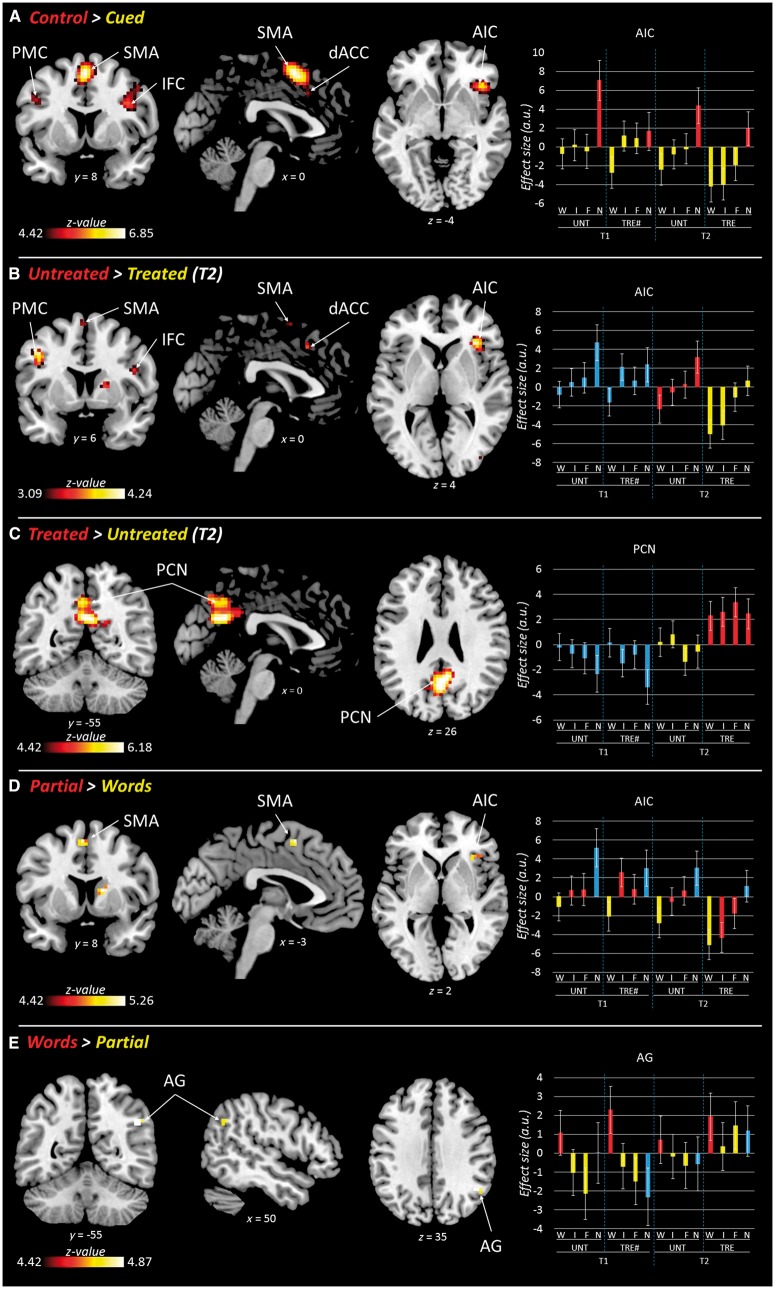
Functional MRI results. (A) Reductions in BOLD response related to immediate facilitation of naming. (B) Reductions in BOLD response related to long-term facilitation of naming. (C) Increased BOLD response for treated (as compared to untreated) items. (D) Activations related to partial (i.e. initial and final phonemes) cues processing. (E) Activations related to whole word cues processing. Results are displayed at *P* < 0.05 (FWE-corr.), except in (B), where they are displayed at *P* < 0.001 (unc.) for cluster extent, without correction at cluster-level (to allow for small-volume correction). Red and yellow bars refer to the conditions compared (red > yellow, as in the title of each contrast). Blue bars are conditions not included in the contrasts. a.u. = arbitrary unit; AG = angular gyrus; AIC = anterior insular cortex; Control = control trials (i.e. noise); Cued = cued trials (i.e. word, initial, and final); dACC = dorsal anterior cingulate cortex; F = final; I = initial; IFC = inferior frontal cortex; N = noise; Partial = partial cues (i.e. initial and final phonemes); PCN = precuneus; PMC = premotor cortex; SMA = supplementary motor area; TRE = treated items (TRE# = ‘to-be-treated' items); UNT = untreated items; W = word; Words = whole word cues; *x y z* = MNI coordinates of brain sections. Sections are displayed in neurological convention.
